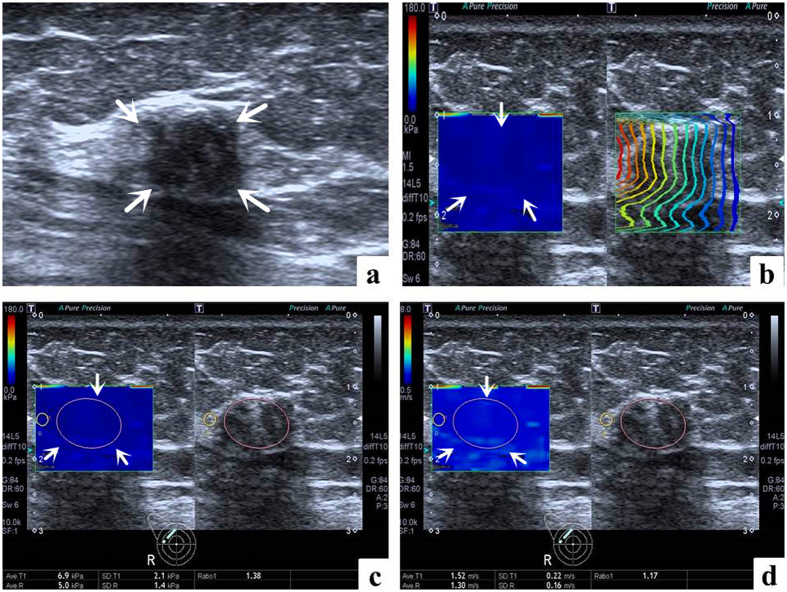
False negative case images in a 68-year-old woman with ductal carcinoma *in situ*. (a) The lesion (arrows) is shown on B-mode ultrasound. (b) The lesion (arrows) shows regularly parallel contour lines on the shear wave propagation mode. (c) The Emean, E_SD_ and Eratio of the lesion (arrows) on elasticity mode are 6.9 kPa, 2.1 kPa and 1.38 respectively. (d) The Emean, E_SD_ and Eratio of the lesion (arrows) on shear wave speed mode are 1.52 m/s, 0.22 m/s and 1.17 respectively.
